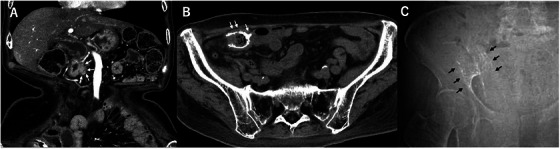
(A, B) Computed tomography (CT) images at 4 months after initiating chemotherapy. (A) The pancreatic tumor (white arrow) shows marked shrinkage, and the duodenal stenosis has improved. (B) The migrated duodenal metallic and biliary plastic stents are in the right lower abdomen (white arrow). (C) Abdominal radiograph obtained 2 weeks after CT shows no change in stent position (black arrow).
